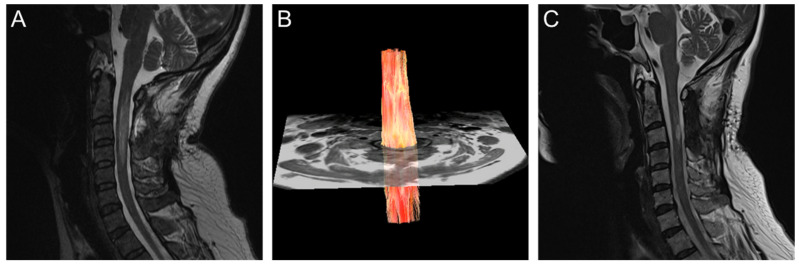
Subsequent MRI study. (A) T2-weighted image (T2WI) showed persistent hyperintensity lesion in C2–C4 spinal cord; (B) diffusion-tensor tractography (DTT) showed intact streamline of white-matter tracts without displacement or interruption; (C) T2WI after 2 months of medical treatment showed residual faint hyperintensity in C2 spinal cord, and focal myelomalacia corresponding to prior excision biopsy in C3 spinal cord.
